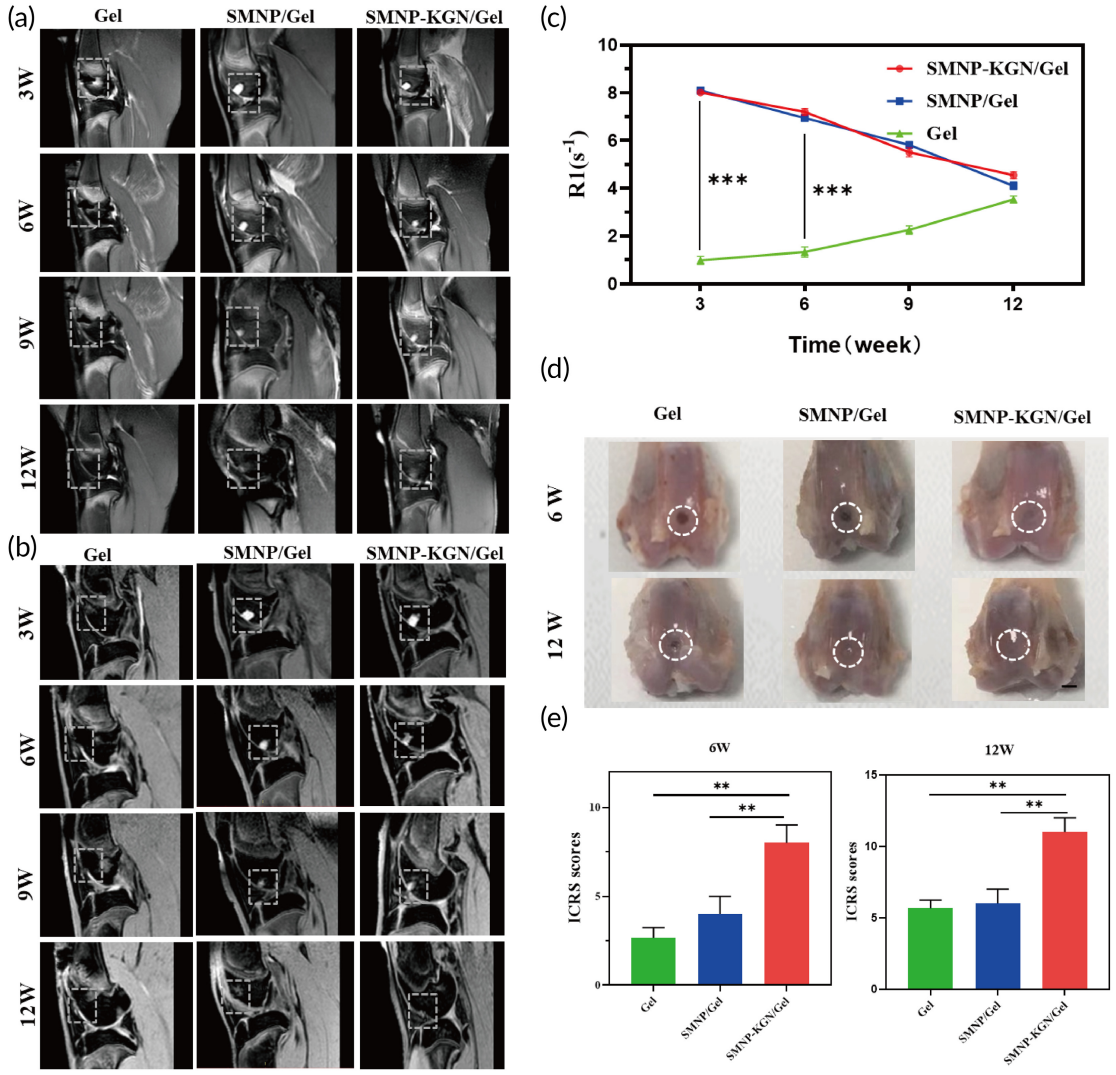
MRI and macroscopic analysis of cartilage defect model. (a) MR proton‐density‐weighted imaging (PDWI) for cartilage evaluation at 3, 6, 9, and 12 weeks after surgery. (b) MR water‐selective cartilage scan (3D_WATSc) for the morphological observation of the degradation of hydrogel. (c) Semiquantitative *R*
